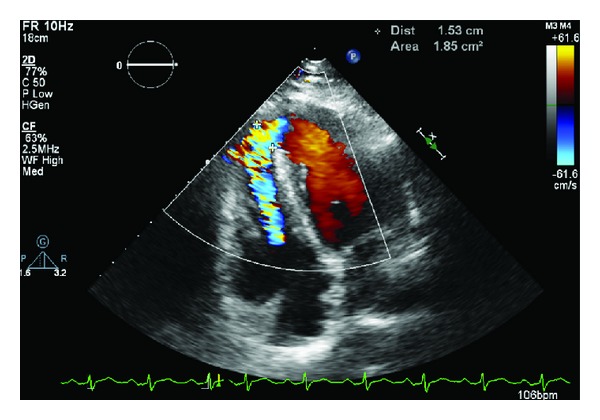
Transthoracic echocardiogram: apical ventricular septal defect with shunt noted.
